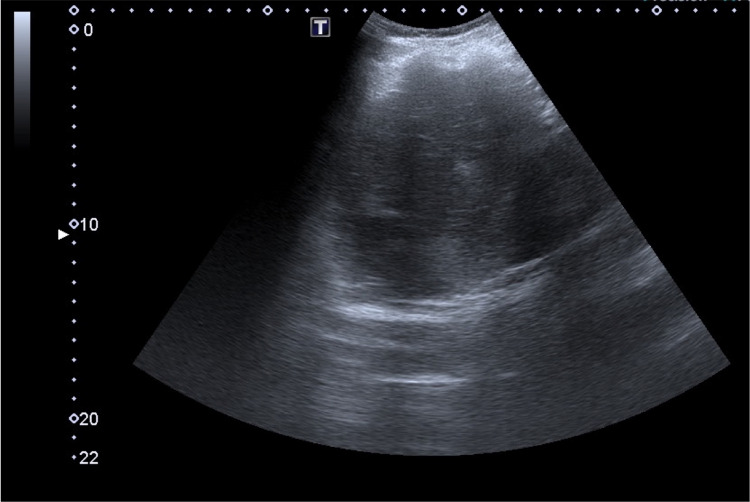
Abdominal ultrasound scan on admission showing spleen with heterogeneous echotexture and poorly defined contours.
